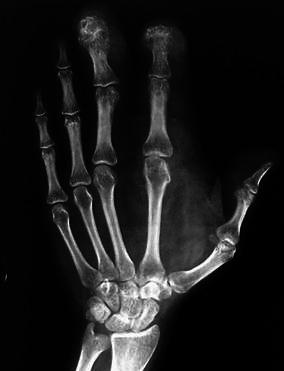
Postoperative plain radiograph (AP view) of the left hand showing partial amputation of the overgrown digits (middle phalanges of the second and third digits have been removed) and reduced soft tissue bulk
